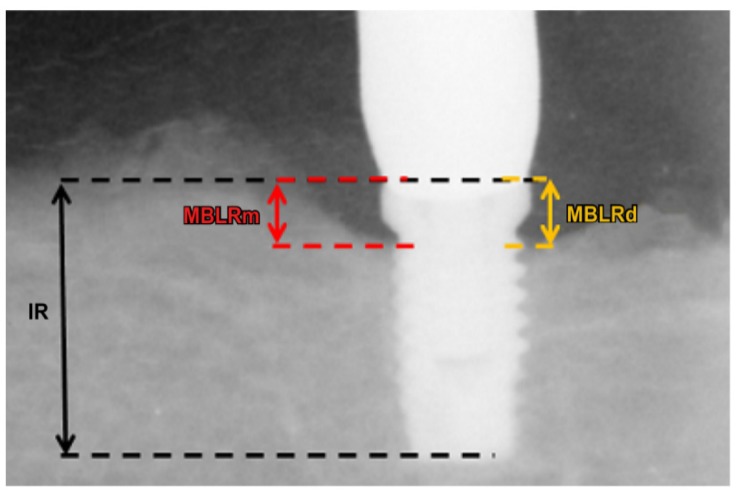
X-ray reference points for measuring vertical marginal bone loss (MBL) around the implant in the mesial aspect (MBLRm) and distal aspect (MBLRd); IR, implant length on the radiograph. Using the length of the actual implant (I), MBL was calculated from the following ratio: I/MBL = IR/MBLR.
